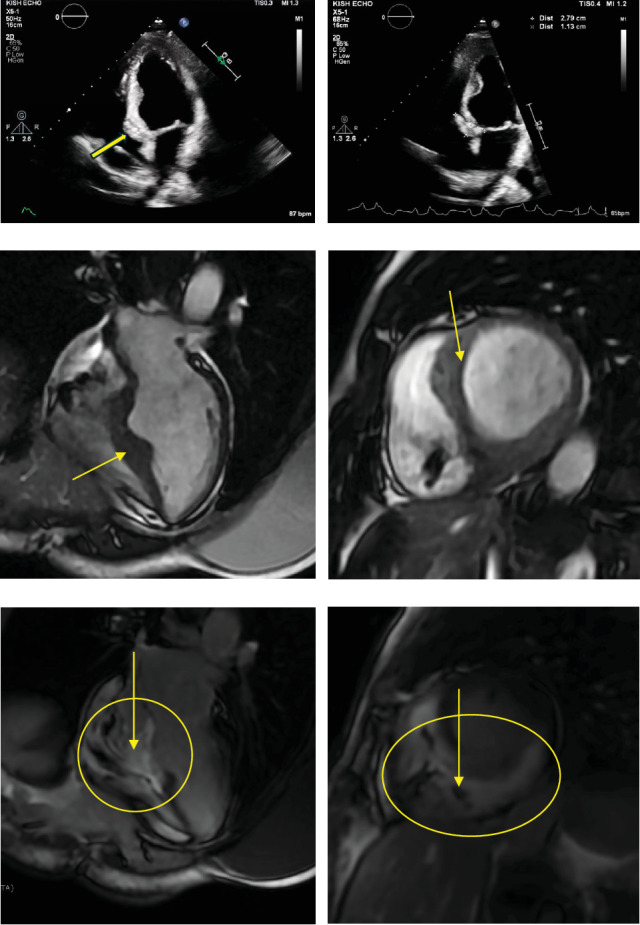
Cardiac mass characterization on transthoracic echocardiography and cardiac MRI. (a, b) Transthoracic echocardiogram performed on initial presentation to our institution: (a) Apical 4-chamber view showing an intracardiac mass at the ventriculo-atrial septal junction. (b) Measuring roughly 2.8 × 1.1 cm. (c–f) Cardiac magnetic resonance imaging for further mass characterization: (c) Four-chamber view demonstrating asymmetric thickening in the interventricular septum and aneurysmal akinetic region at the basal inferoseptum (yellow arrow). (d) Short-axis view with asymmetric septal thickening within the interventricular septum (yellow arrow). (e) Postgadolinium four-chamber view revealing dense late gadolinium enhancement (LGE) involving basal to mid septum and inferior wall, with extension into the anterior wall. (f) Postgadolinium short-axis view showing dense LGE and central hypointensity in the inferoseptum, consistent with necrosis (yellow arrow).
